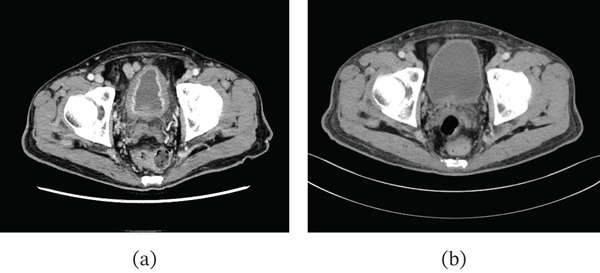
CT findings. (a) CT at the onset of irritative bladder symptoms revealed edematous changes in the bladder wall. (b) After treatment with prednisolone, CT findings showed significant improvement in the bladder wall.
